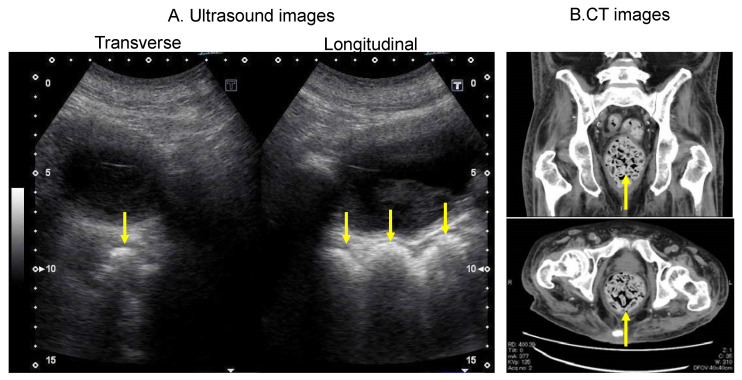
Ultrasound images of a patient who had frequent episodes of diarrhoea with intense faecal impaction in the rectum on CT imaging. (A) Rectal ultrasound images showing a hyperechoic area indicating faecal retention (arrows). (B) CT image showing faecal retention in the rectum (arrows). The upper image shows a longitudinal section, and the lower image shows a transverse section. CT, computed tomography.
